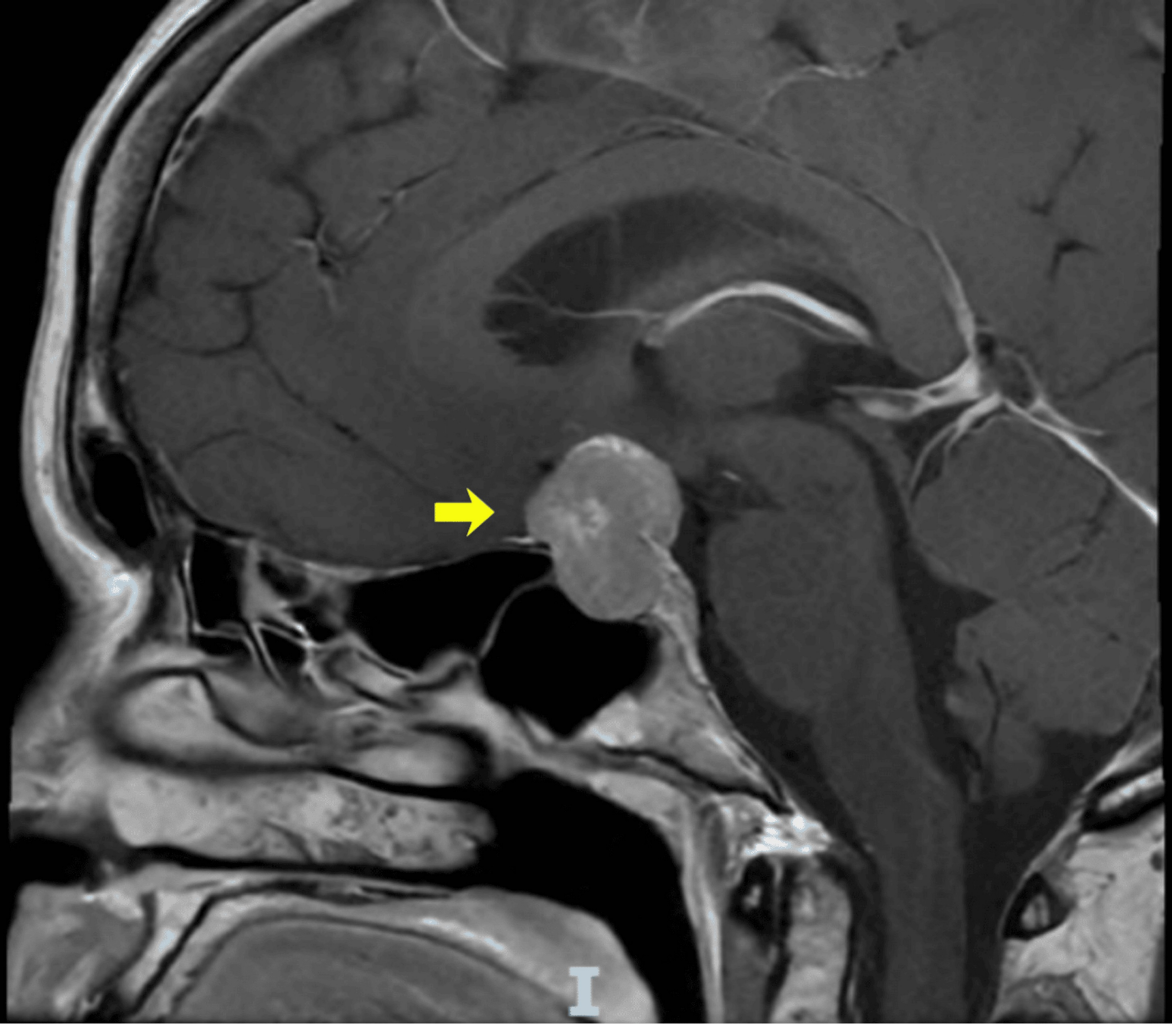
Sagittal T1-weighted contrast-enhanced magnetic resonance imaging Sagittal T1-weighted contrast-enhanced magnetic resonance imaging showing a sellar mass measuring 19 × 31 × 32 mm, appearing isointense on T1-weighted sequences with heterogeneous contrast enhancement and suprasellar extension, causing the compression of the optic chiasm (yellow arrow)
